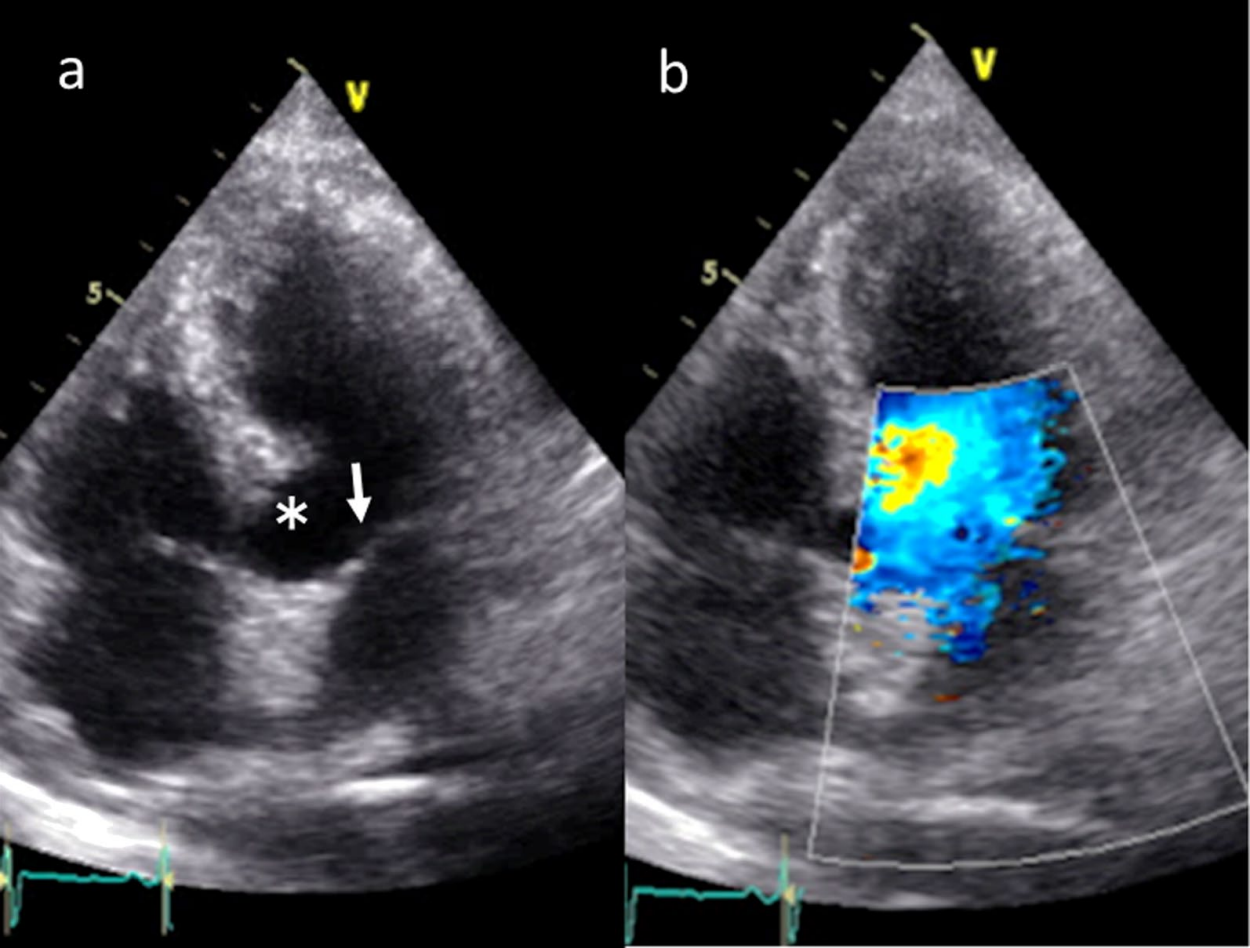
Postoperative transthoracic echocardiographic views. a No evidence of systolic anterior motion (white arrow) and resected part of the basal interventricular septum (white asterisk). b Color Doppler imaging of no mitral regurgitation
